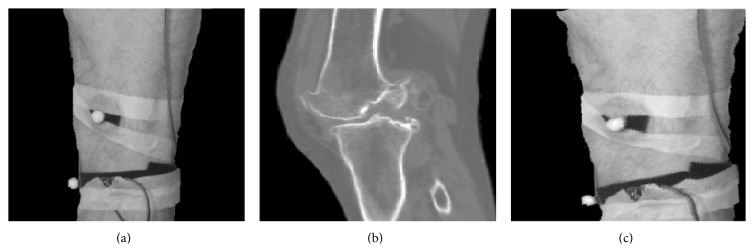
Registration infrared image and CT image. (a) Infrared camera image; (b) CT image; (c) registered image.
